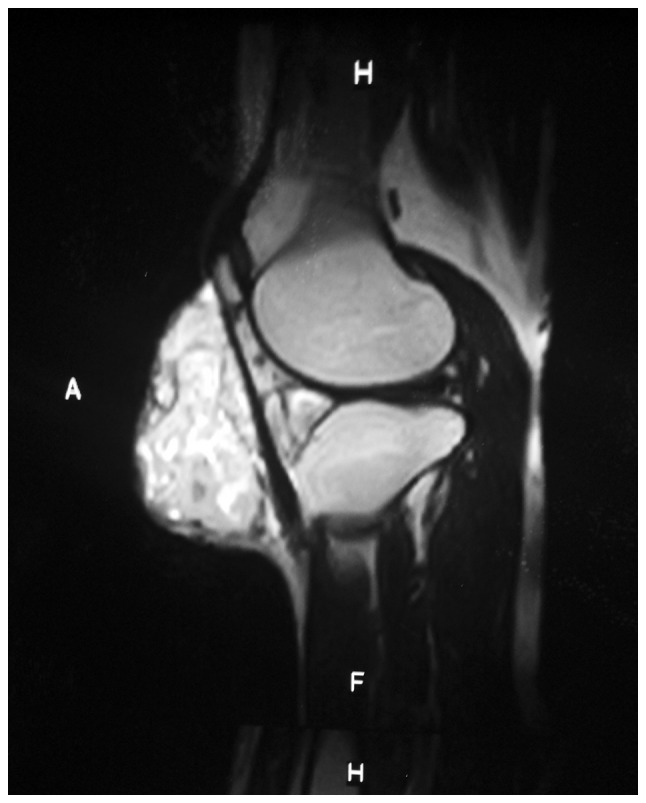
T1 fat-suppressed contrast-enhanced magnetic resonance imaging revealed a hyperintense central section and hypointense irregular wall, corresponding to a myxoid and fibrous pattern.
